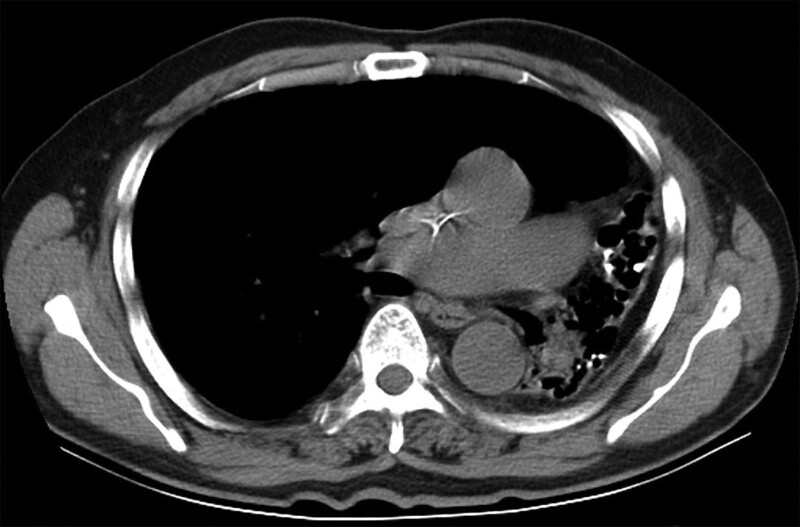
Chest CT image showing significantly decreased volume and bronchiectasis of the left lung corresponding to pulmonary hypoplasia and compensatory expansion of the volume of the right lung. CT = computed tomography.
